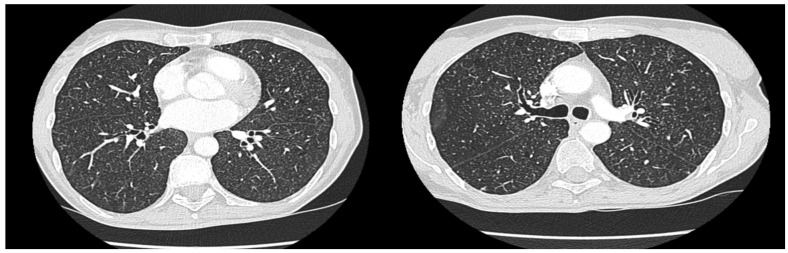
Chest CT showing micronodular lesions consistent with miliary TB.
